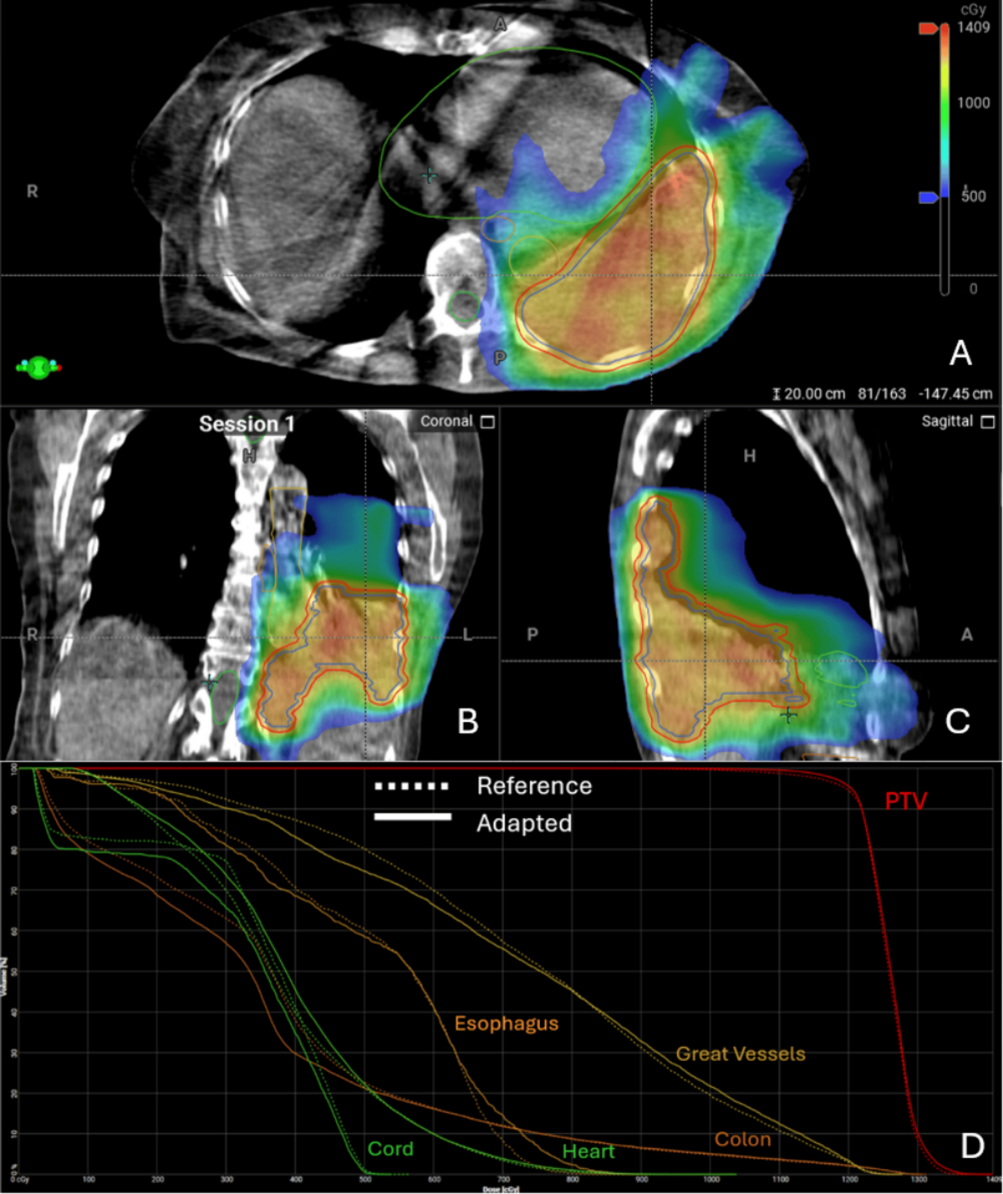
Second Fraction of Online Adaptive Radiotherapy to a Bulky Thoracic and Abdominal Mass Cone-beam computed tomography (CBCT) and dose-volume histogram (DVH) from the second fraction of adaptive stereotactic body radiotherapy (SBRT). Dose colorwash with relevant target volumes and avoidance structures contoured on Ethos platform (Varian Medical Systems, Inc., Palo Alto, CA, USA) on axial (A), coronal (B), and sagittal (C) views. DVH (D) for both the selected adapted plan (solid line) and reference plan (dashed line) for planning target volume (PTV; red), great vessels (gold), colon (orange), esophagus (brown), heart (light green), and spinal cord (dark green).
